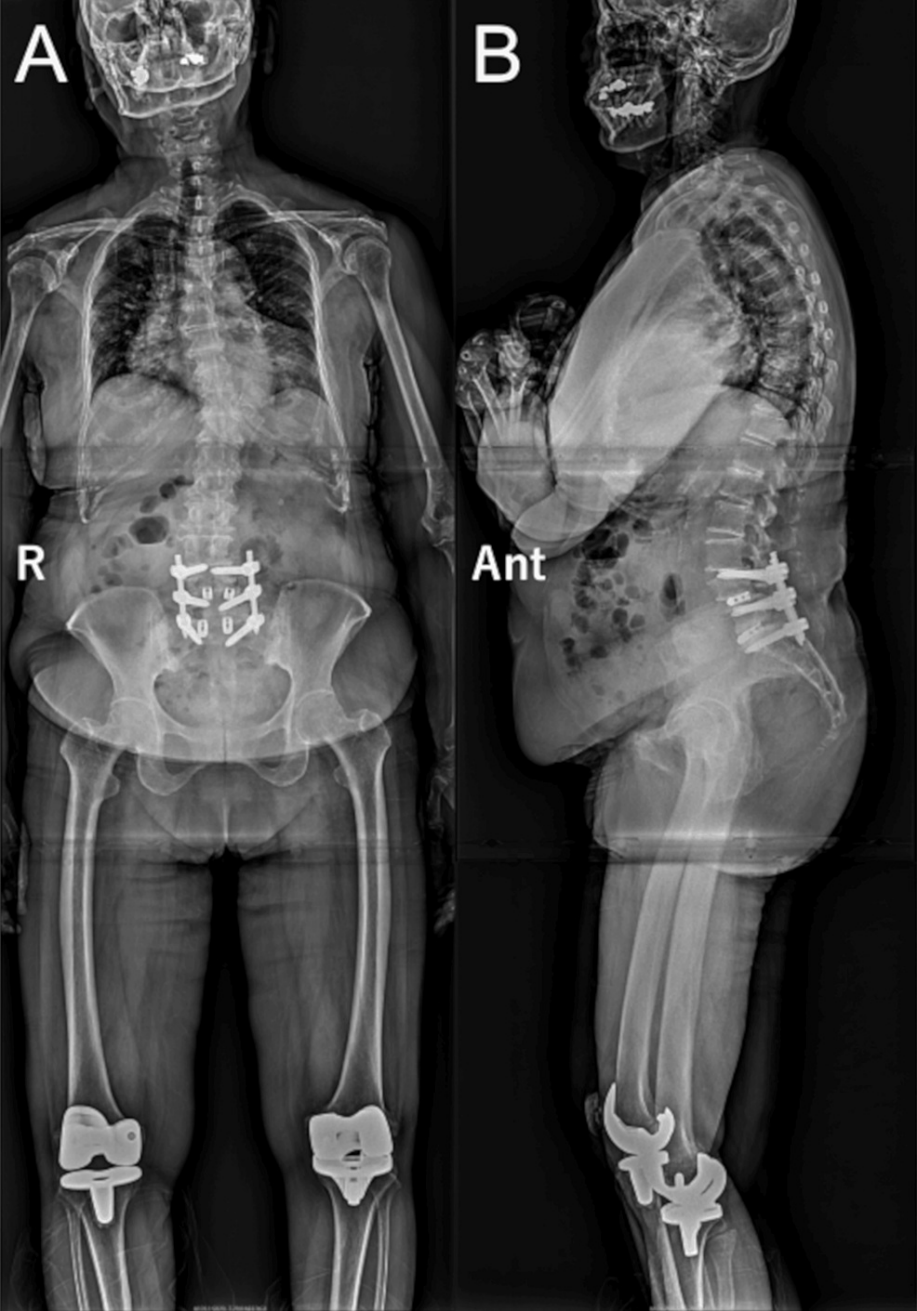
Radiographic images after the first operation A: anterior-posterior projection; B: lateral projection; posterior lumbar intervertebral fusion from L4 to S1 is performed (Ant: anterior, r: right).
